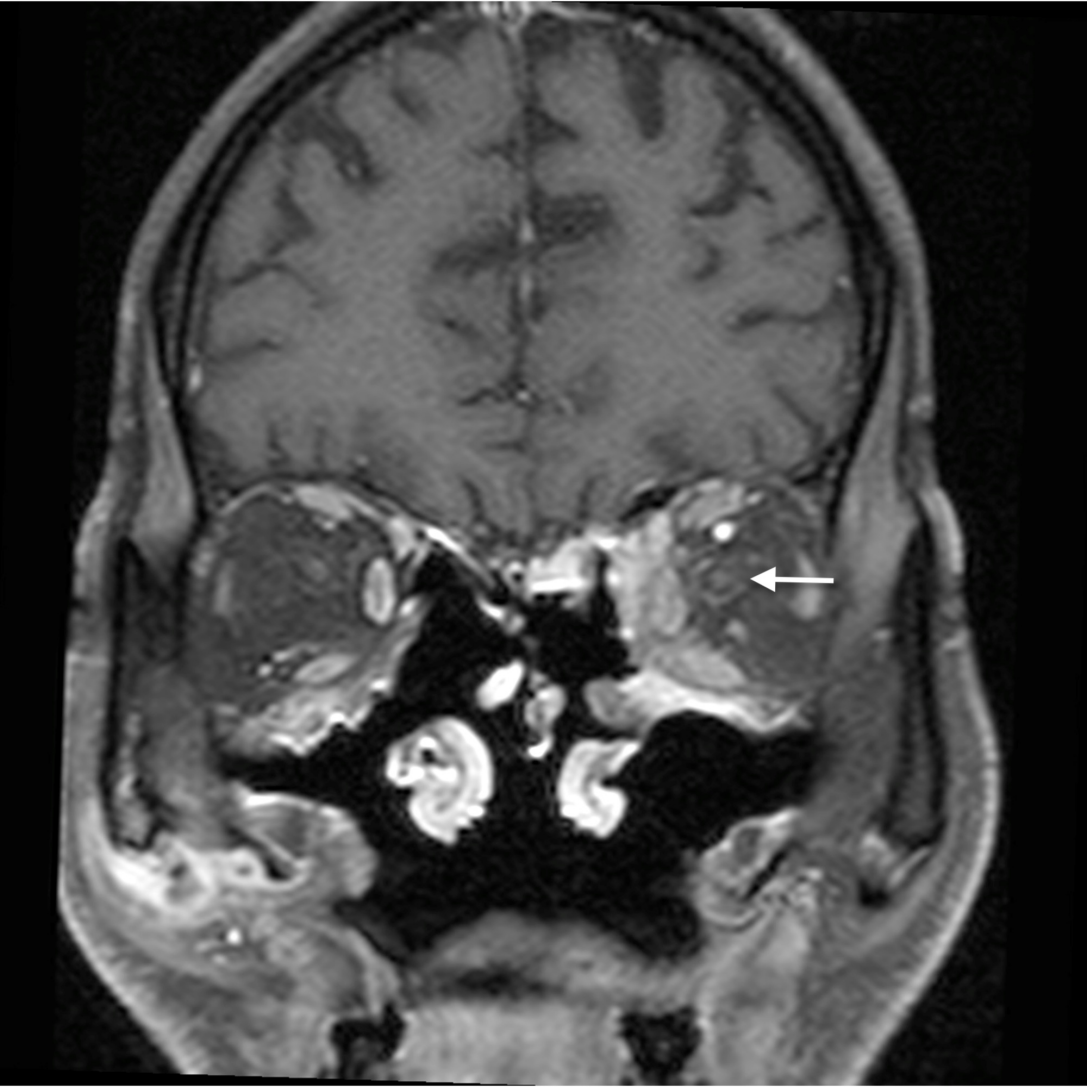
Optic perineuritis: coronal post gadolinium T1WI shows mild perineural enhancement around the left optic nerve (arrow), indicative of optic perineuritis. Enhancement is also noted in the extraconal compartment medially, suggestive of orbital cellulitis T1WI - T1-weighted image
